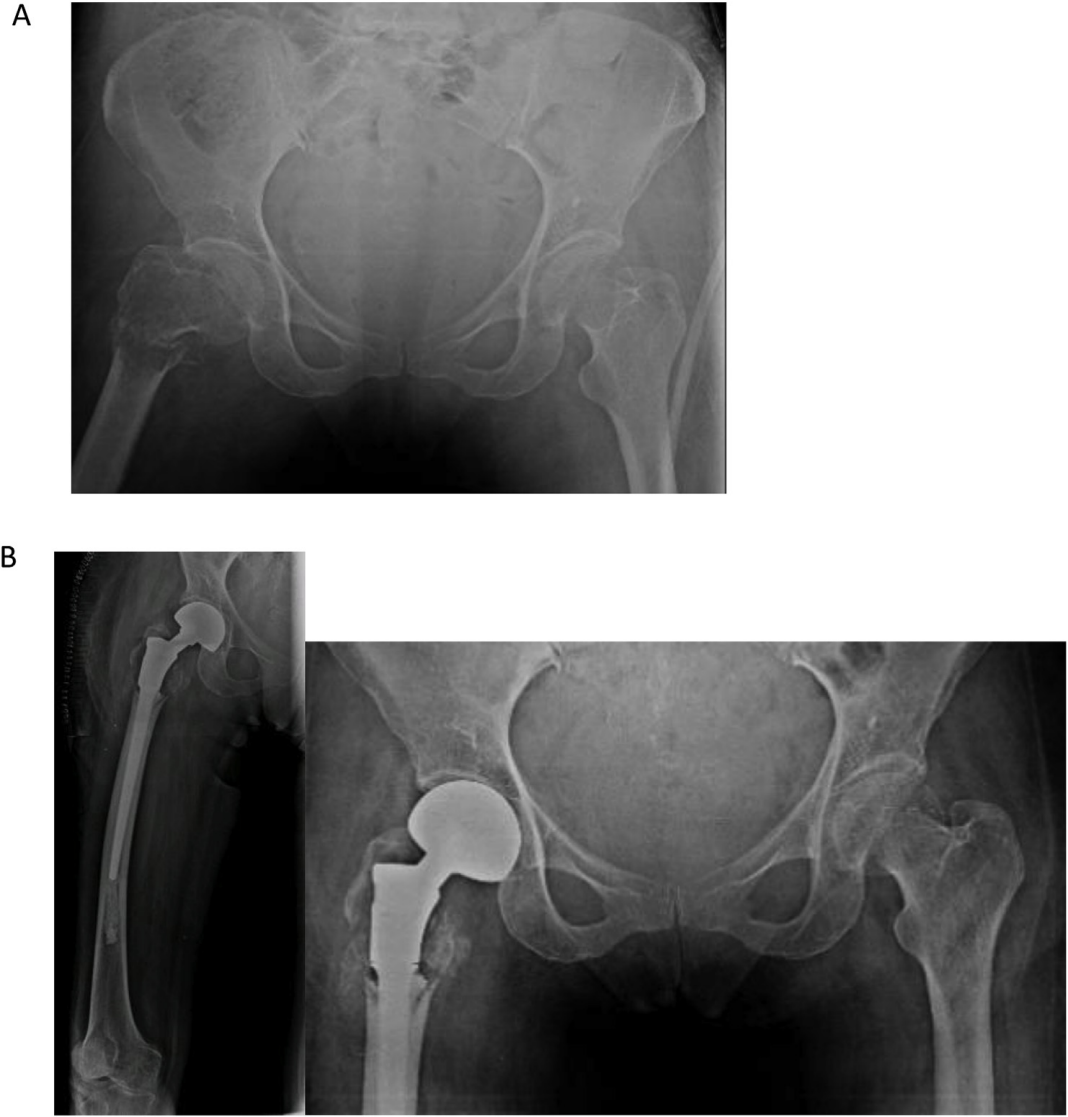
55 years old female known case of metastatic Carcinoma endometrium with metastases to lungs, bones and peritoneum presented with right leg pain. A: X-ray Pelvis showing lytic lesion in the proximal Femur with pathological fracture. B:Palliative surgery was performed for pain relief and nursing care, right sided Proximal Femur Replacement with Bipolar hemiarthroplasty.
